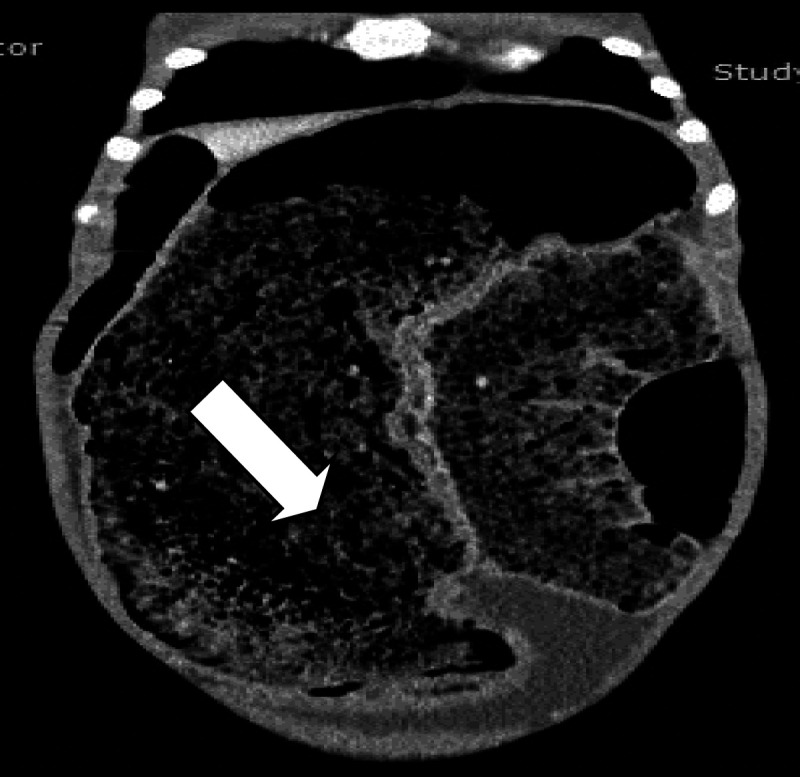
Contrast-enhanced frontal CT section showing extended fecaloma
